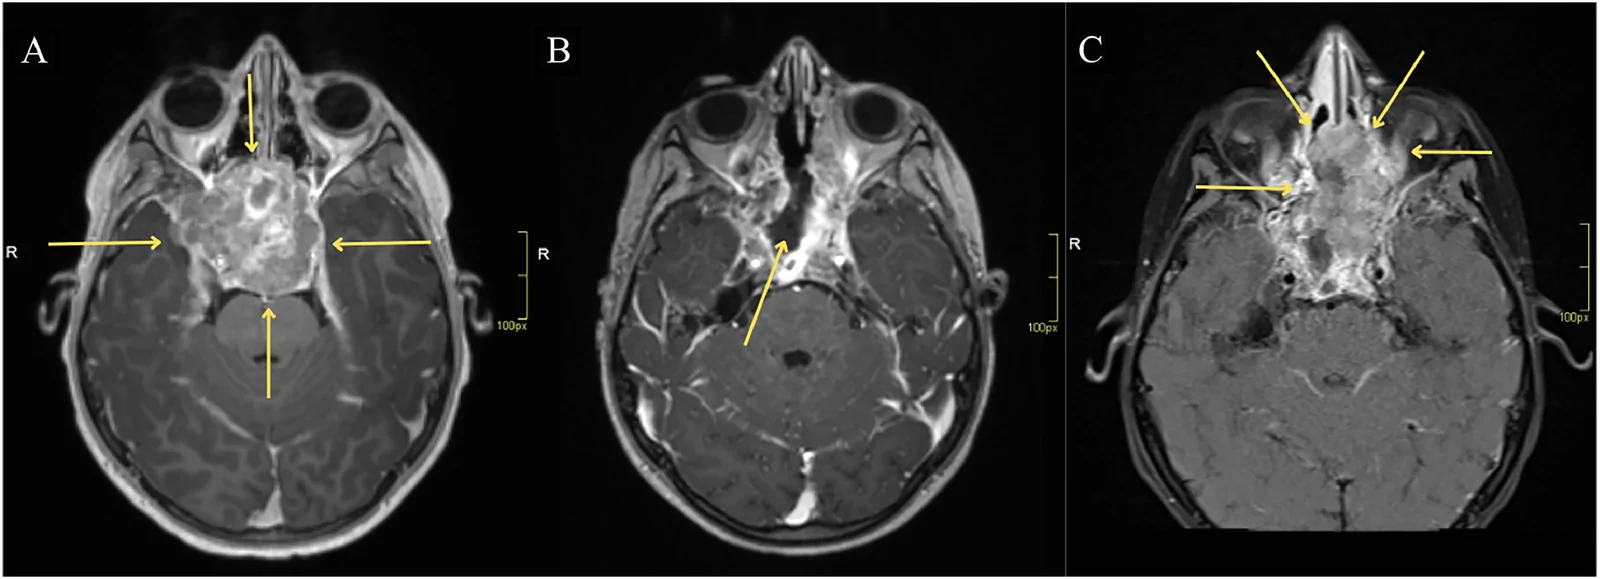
Interval progression of SMARCB1-deficient sinonasal carcinoma despite multimodal treatment. (A) Initial axial contrast-enhanced T1-weighted image demonstrates a large central skull base mass (arrows). (B) Postoperative axial contrast-enhanced T1-weighted image demonstrates a central surgical cavity within the mass following tumor debulking (arrows). (C) Subsequent axial contrast-enhanced T1-weighted image demonstrates interval tumor regrowth with increased anterior extent (arrows).
